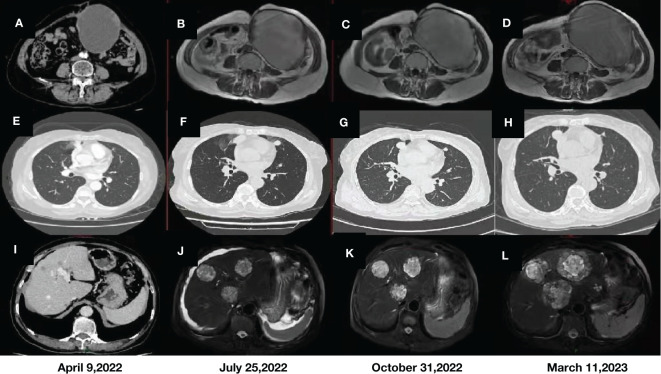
CT and MRI images of the anterior abdominal wall mass (A, B) and liver metastases (I, J) before immunotherapy. MRI images of the anterior abdominal wall mass (C, D) and liver metastases (K, L) after immunotherapy. Comparison of CT images of metastatic tumors in both lungs before (E, F) and after immunotherapy (G, H).
